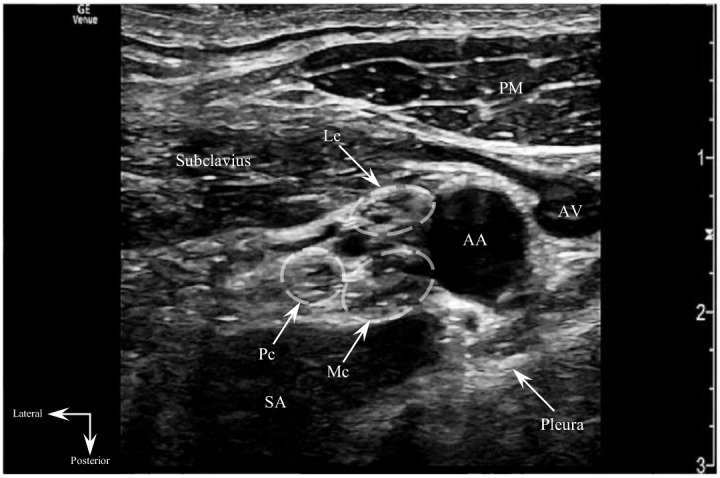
Transverse sonogram of the costoclavicular space depicting the relevant sonoanatomy for costoclavicular brachial plexus block performance. The brachial plexus cords (dashed ovals) are shown lying lateral to the axillary artery and between the intermuscular plane composed of the subclavius and serratus anterior (upper slips) muscles. Note the cords are tightly clustered in a relatively superficial location lateral to the axillary vessels, making the costoclavicular space an ideal target site for brachial plexus blockade. *AA*, axillary artery; *AV*, axillary vein; *Lc*, lateral cord; *Mc*, medial cord; *Pc*, posterior cord; *PM*, pectoralis major; *SA*, serratus anterior.
